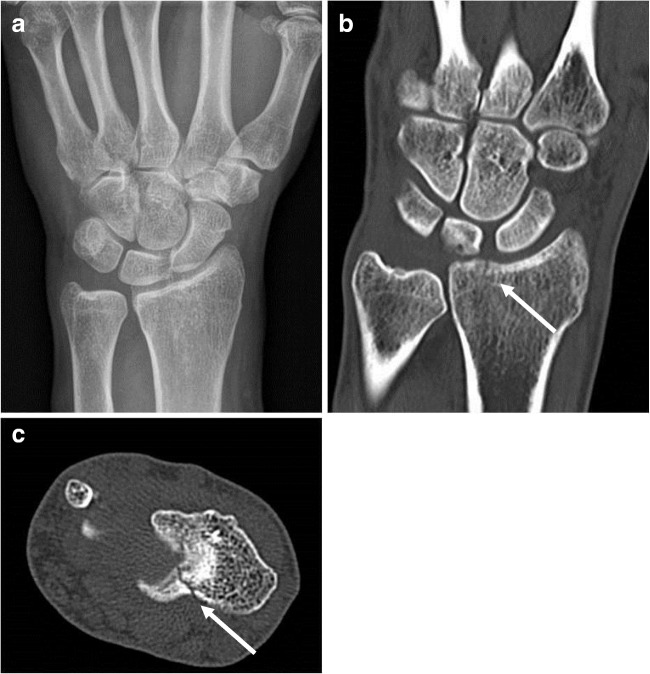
Missed fracture on CR with treatment upgrade after CT. A 45-year-old male with a painful left wrist after fall on an outstretched arm and with swelling at the level of the lunate, but no hematoma or pressure pain. CR was negative for fractures (a). Based on these images, both clinicians and the observers proposed conservative treatment with a pressure dressing. CT demonstrated an intra-articular, distal radius fracture (*arrows*, b and c). All observers upgraded to cast treatment. The patient was finally treated with cast for 3 weeks
